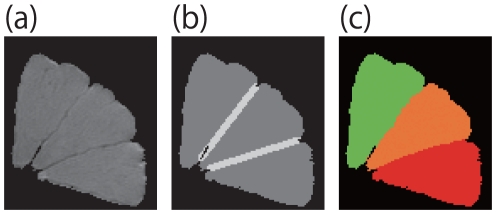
Sections of orange (a) is the input CT image. The white areas in (b) are the ROIs. (c) is the result of the proposed method.
